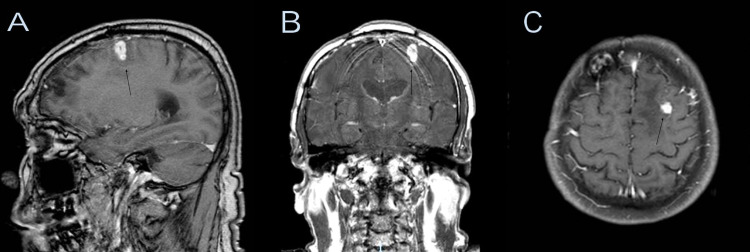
Initial brain MRI with and without contrast showing an intra-axial enhancing mass (black arrow) with associated edema measuring 0.9 x 0.8 x 1.7 cm at the left posterior frontal lobe. Sagittal (A), coronal (B), axial (C).
